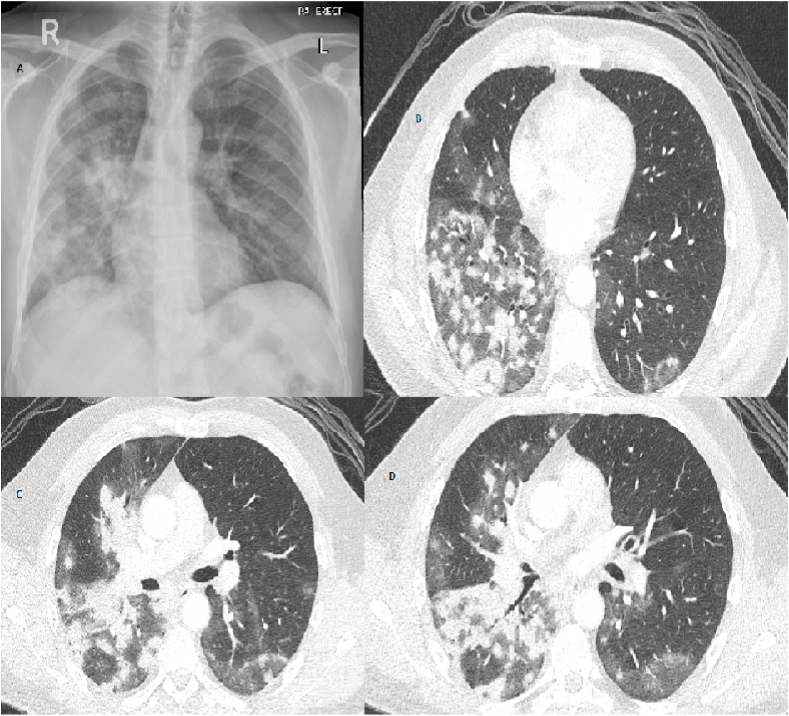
1A Chest X-ray Image showing inhomogeneous patchy infiltrates in the right mid and lower zones. (1B,C,D) CT chest Image showing multiple nodules in the right lower lobe with patchy ground-glass opacifications.
